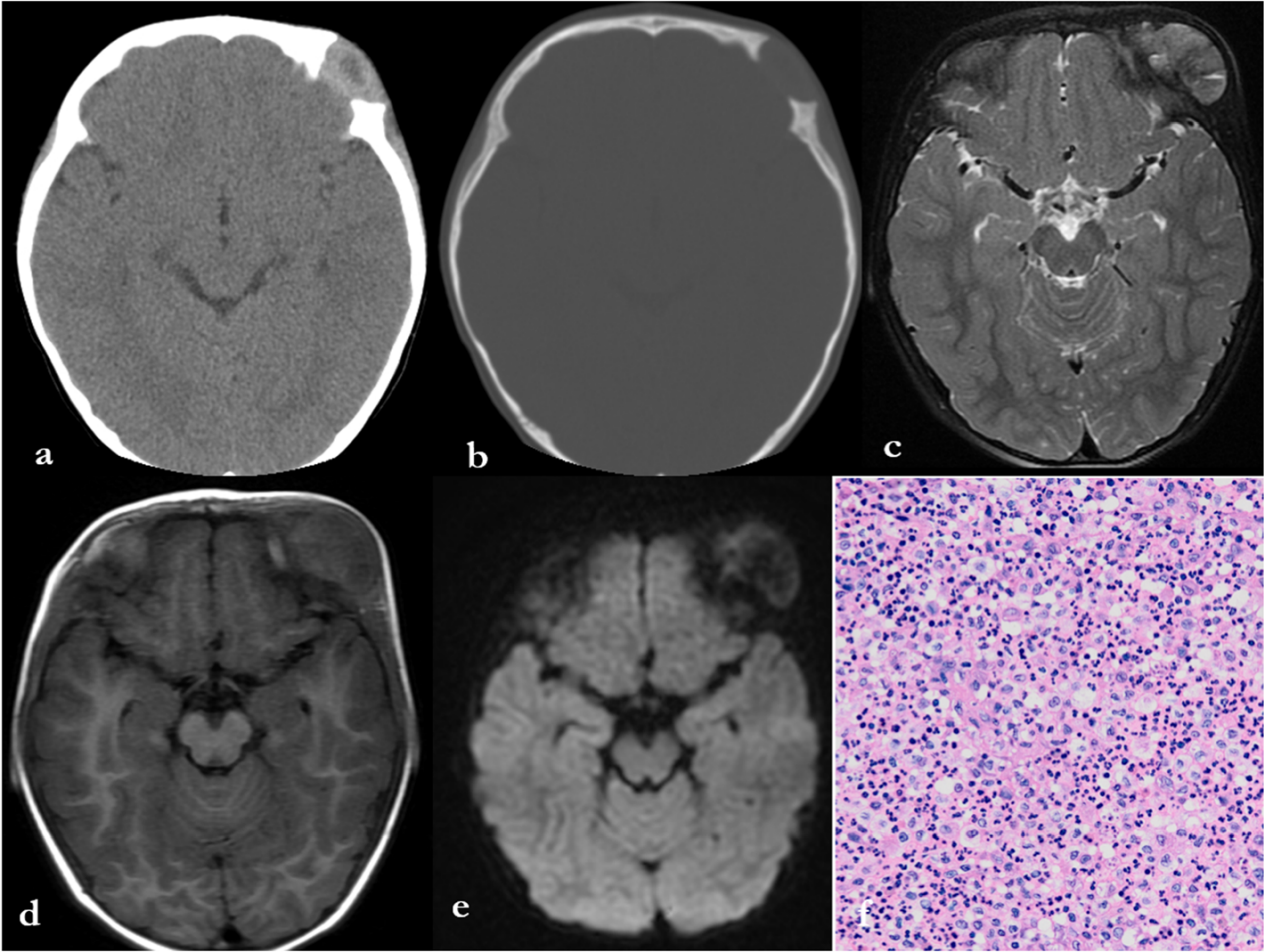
Axial computed tomography of a 2-year-old male patient (a, b) showing bevelled-edge appearance in the left frontal bone. Axial MR images show hyperintensity on T2WI (c), hypointensity on T1WI (d), and slight hypointensity on diffusion weighted imaging (e). The diagnosis of LCH was confirmed by pathology after surgery (pathological slice HE × 200) (f)
